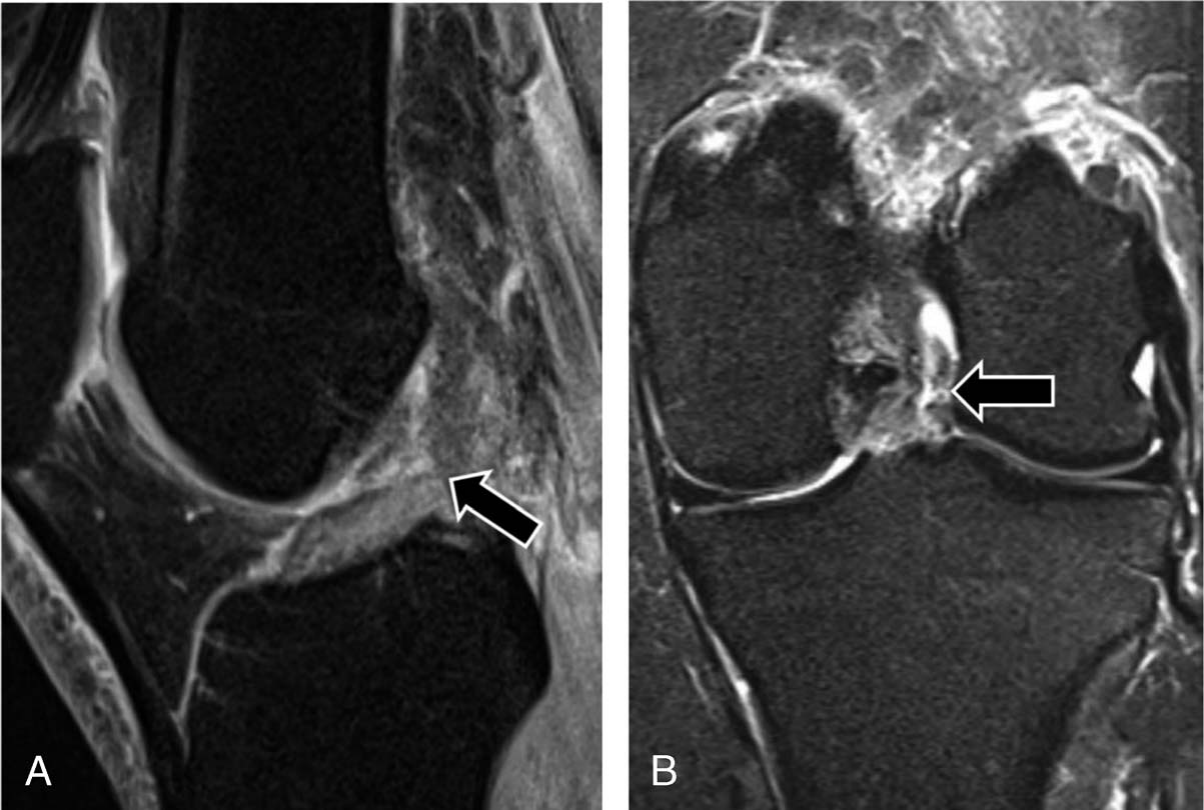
MRI of the left knee of a 41-year-old woman with knee injury 1 week earlier. Sagittal intermediate-weighted turbo spin echo image with fat suppression (A) and coronal short tau inversion recovery image (B) show a full-thickness tear of the midsubstance of the ACL (arrows), which was confirmed by arthroscopic surgery. The DCNN and all 3 radiologists correctly diagnosed the full-thickness ACL tear.
